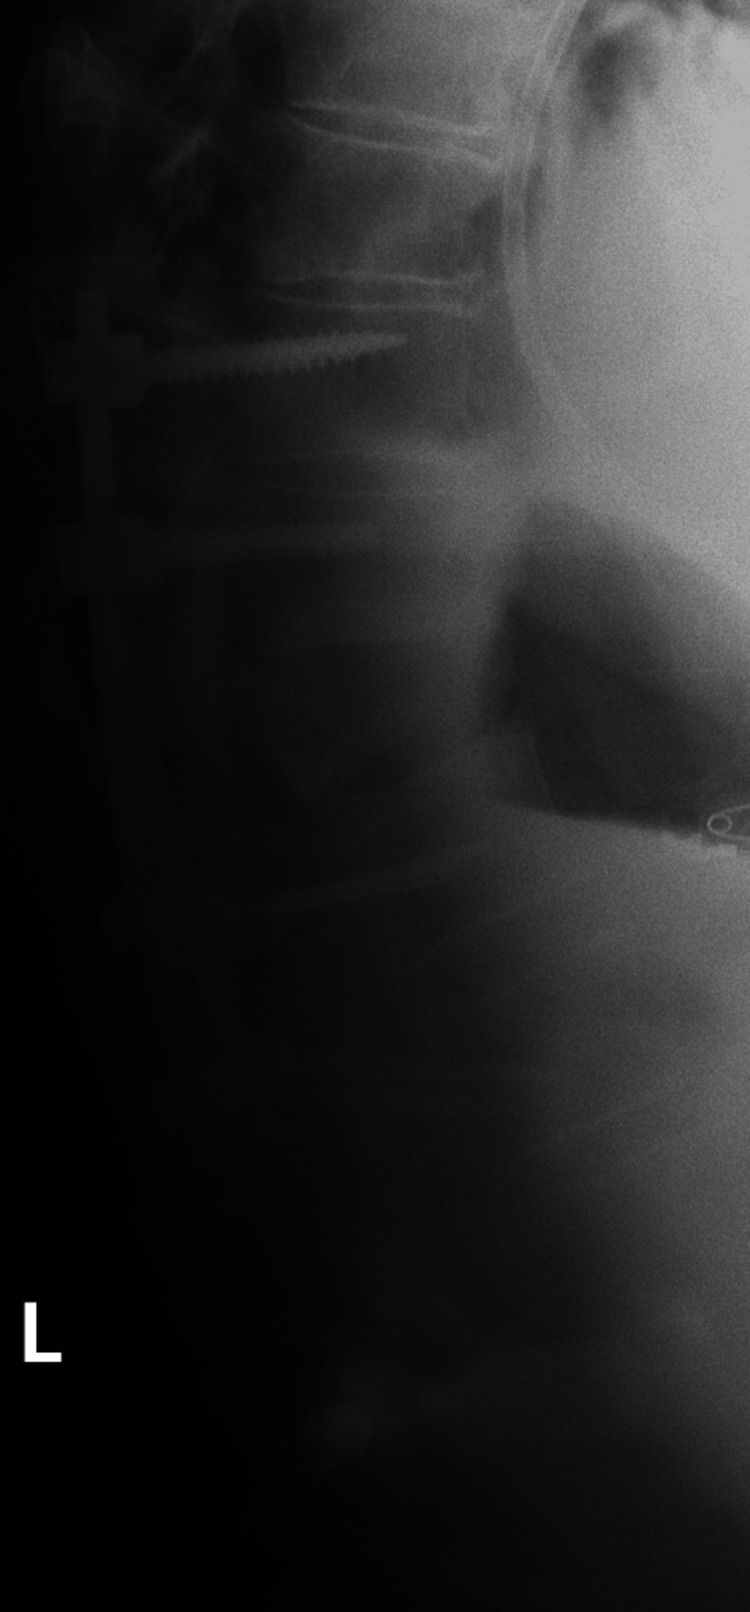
Radiograph of the thoracic spine. Radiograph of the thoracic spine of this 79-year-old man, acquired four weeks postoperatively, demonstrating lucency in the anterior mid-vertebral body of T9, consistent with the patient’s known T9 vertebral body reverse Chance fracture, and T10-L2 fusion hardware surrounding the T12 pathologic compression deformity.
